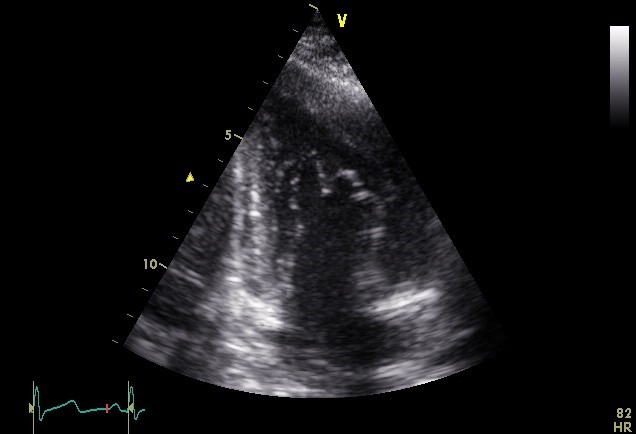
The patient was just prescribed low dose diuretic (Furosemide 20 mg daily) and advised to annually follow up assessment that is continuing till now, for about two years without new changes in clinical or echocardiographic findings.
